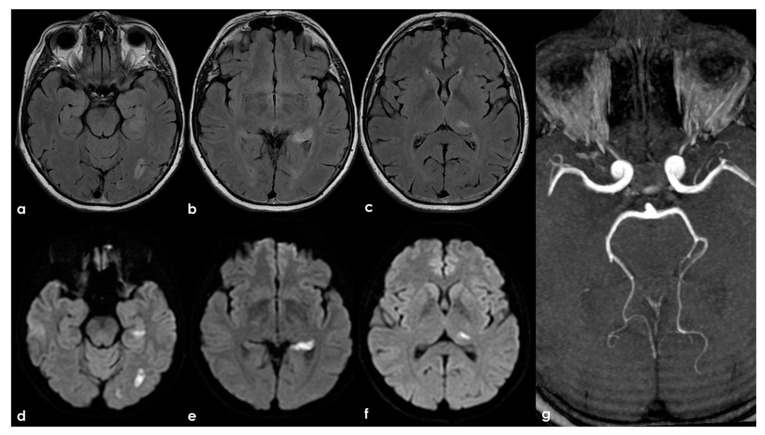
Brain MRI (axial FLAIR in panels (a–c) and the corresponding DWI slices in panels (d–f)) showing multifocal ischemic lesions in the left PCA territory with a patent PCA on MRA (panel (g)).
